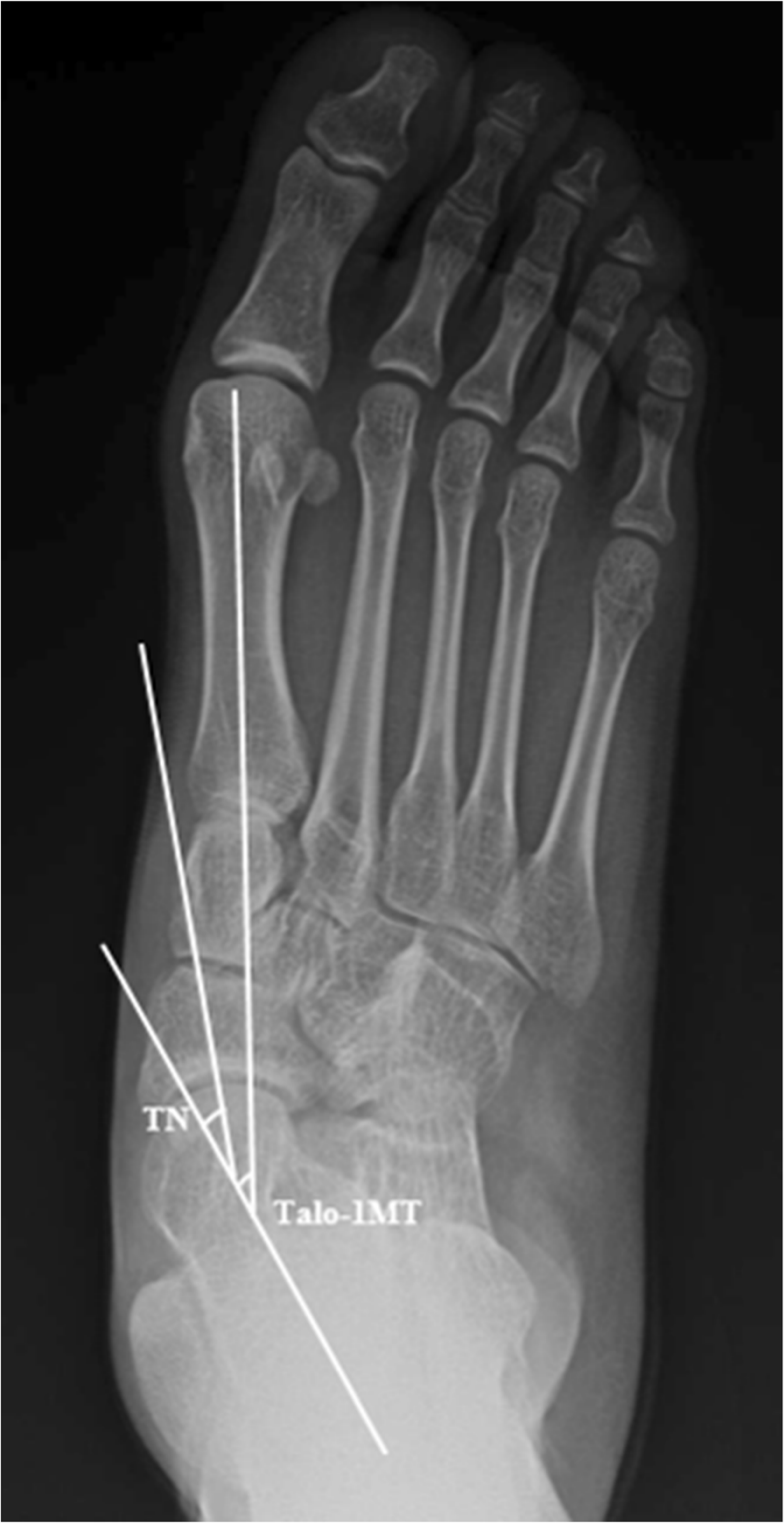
On weightbearing anteroposterior (AP) foot radiographs, the AP talus-first metatarsal angle (AP talo-1MT) and talonavicular coverage angle (TN) are measured
